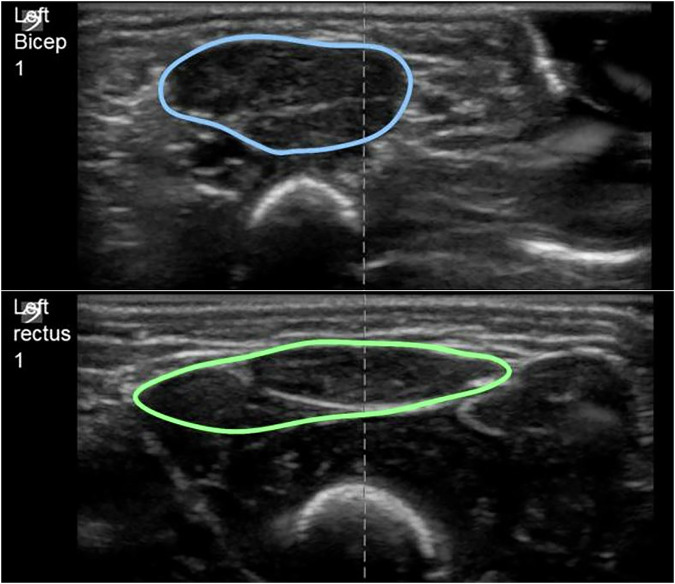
Ultrasound measurement of upper and lower extremity muscle cross-sectional area. Representative ultrasound images of the biceps (upper panel) and rectus femoris (lower panel) demonstrating muscle cross-sectional area aquisition. Images were obtained using a linear probe positioned perpendicular to the muscle belly, including the entire muscle contour and the intramuscular tendon seen as an echogenic band in the center of the muscle belly.
